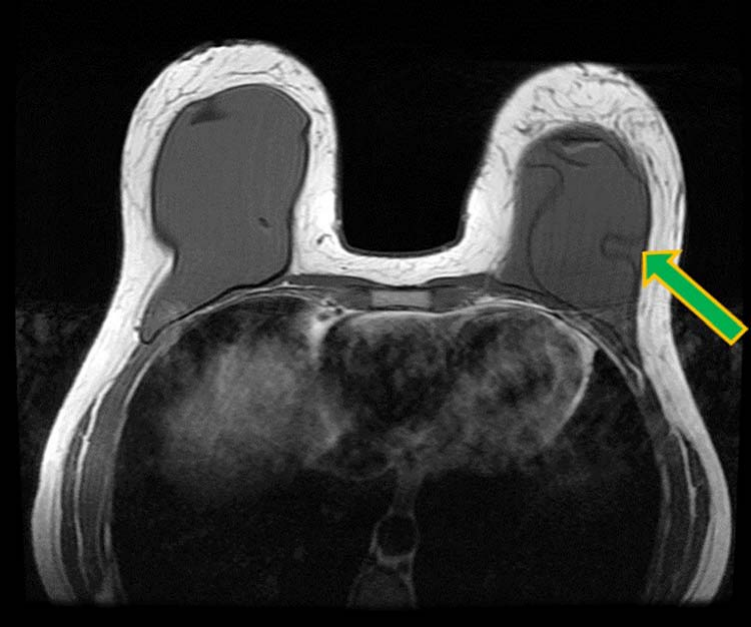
Ruptured left breast implant on MRI as demonstrated by the linguine sign (indicated by green arrow).
